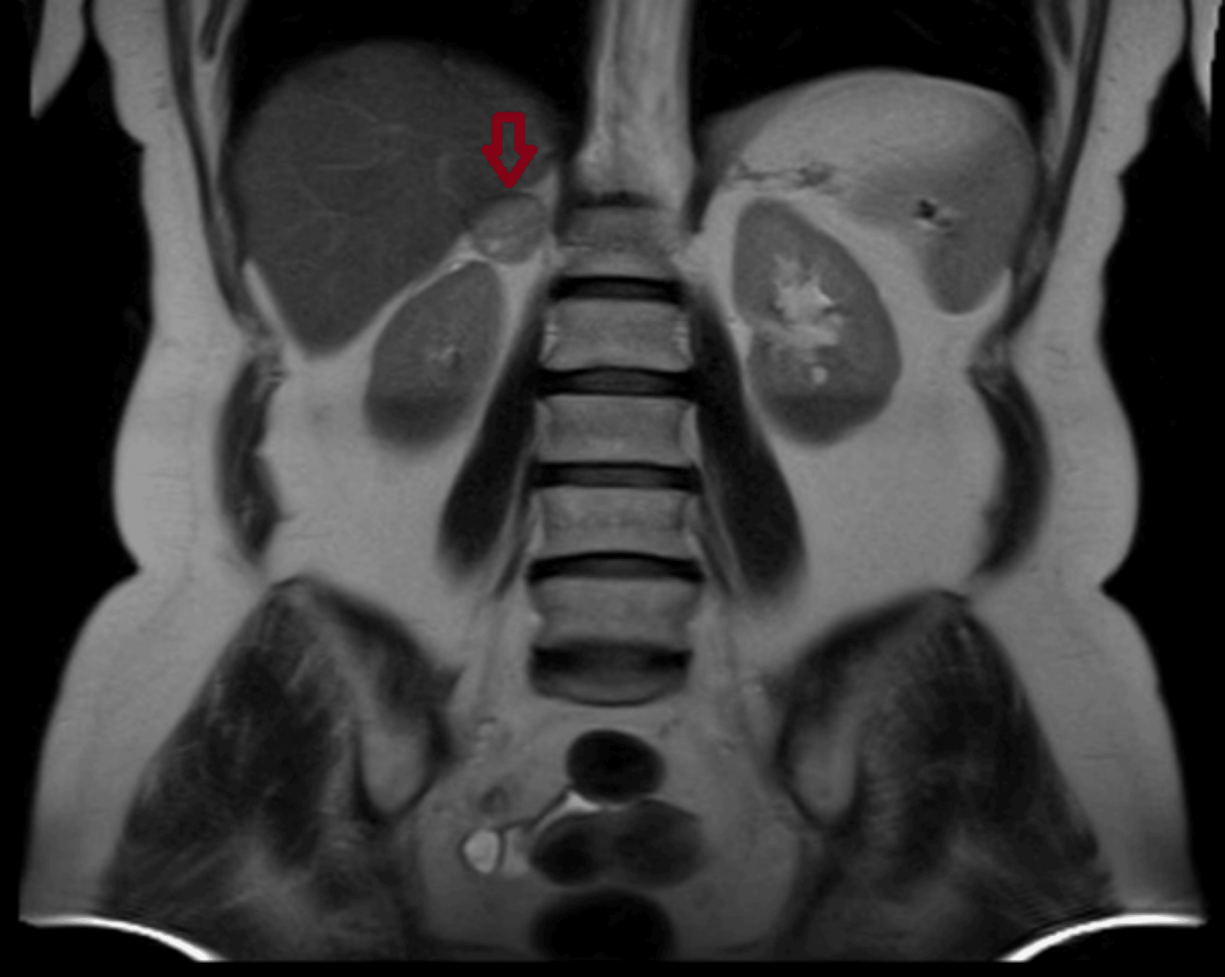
Adrenal imaging findings of Patient 4 An abdominal MRI (coronal plane) of a 57-year-old woman with a right adrenal incidentaloma measuring 30 mm.
